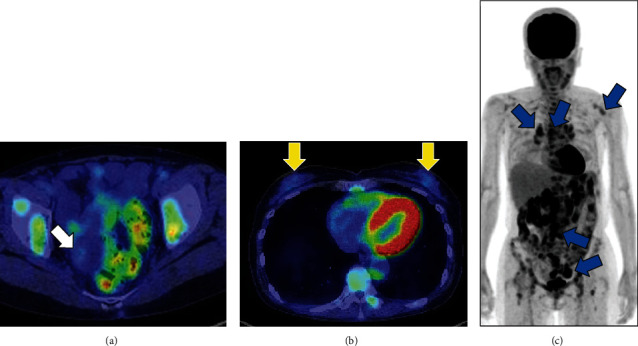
PET-CT findings. (a) PET/CT shows moderate FDG accumulation in the nodule of the right ovarian tumor (white arrow). (b) No significant FDG accumulation can be observed in mammary glands (yellow arrows). (c) Abnormal FDG accumulation can be observed in the lymph nodes of the supraclavicular, mediastinum, hilar, para-aorta, and pelvic regions (blue arrows). PET/CT: positron emission tomography/computed tomography; FDG: F-18 fluorodeoxyglucose.
